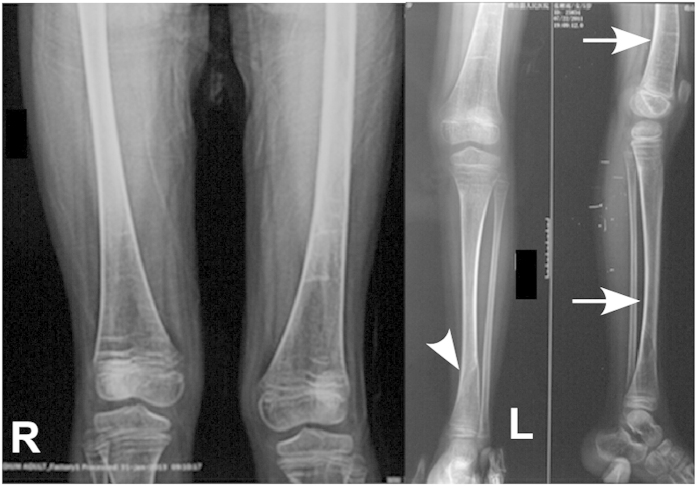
Radiographic evaluation for patient 1. Arrowhead: fracture in left femur. Arrow: deformity of left shinbone and femur.
